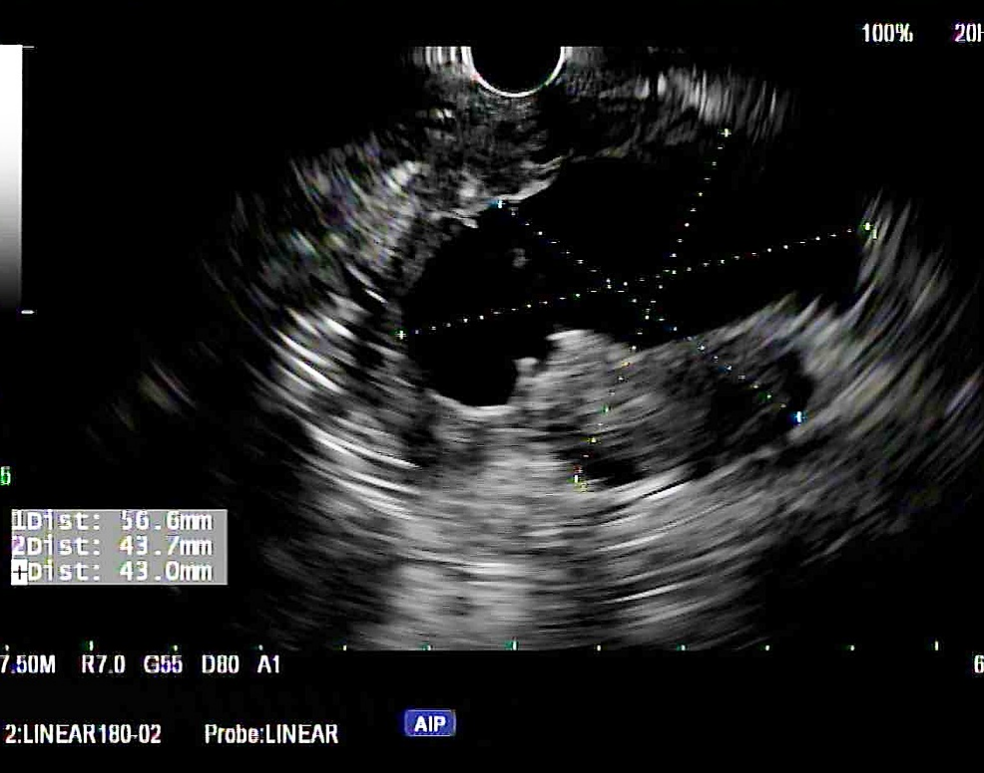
Endosonography (EUS) image of pancreatic lesion
